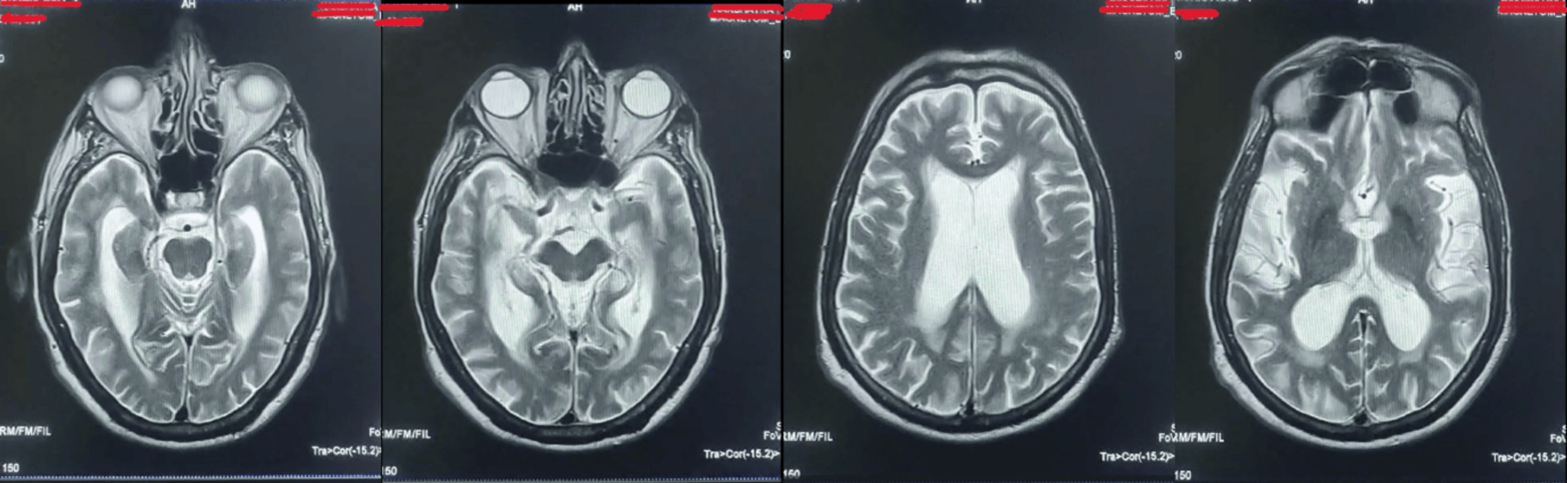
CT scan images showing normal lateral ventricles.
